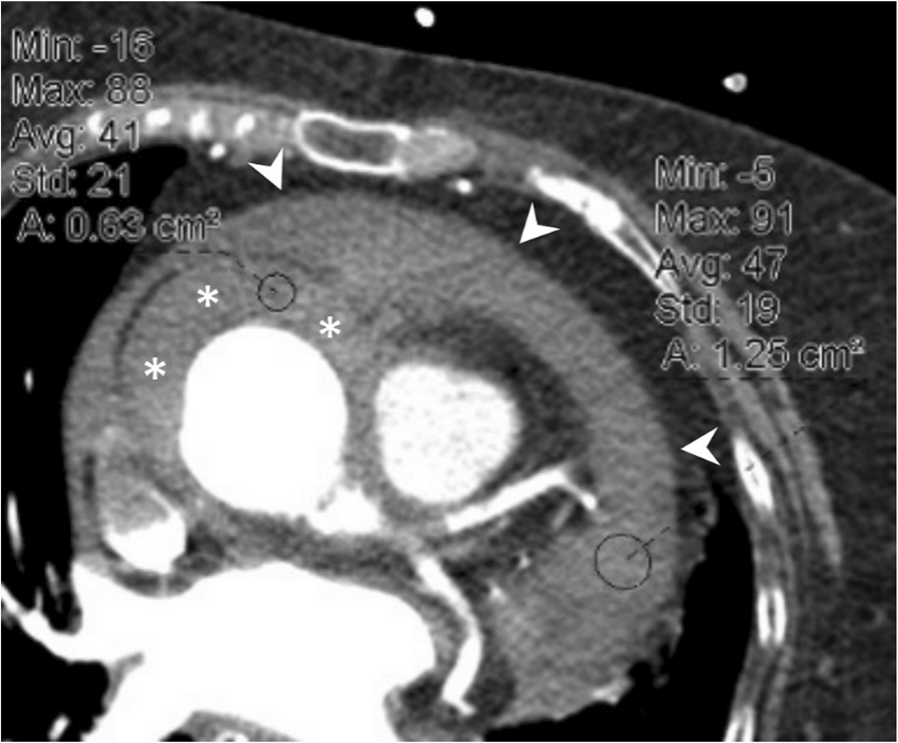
A 86-year-old woman with acute onset of pain in the chest. Axial contrast-enhanced CT image reveals hemopericardium (arrowheads) and intramural hematoma of the ascending aorta (asterisks)
